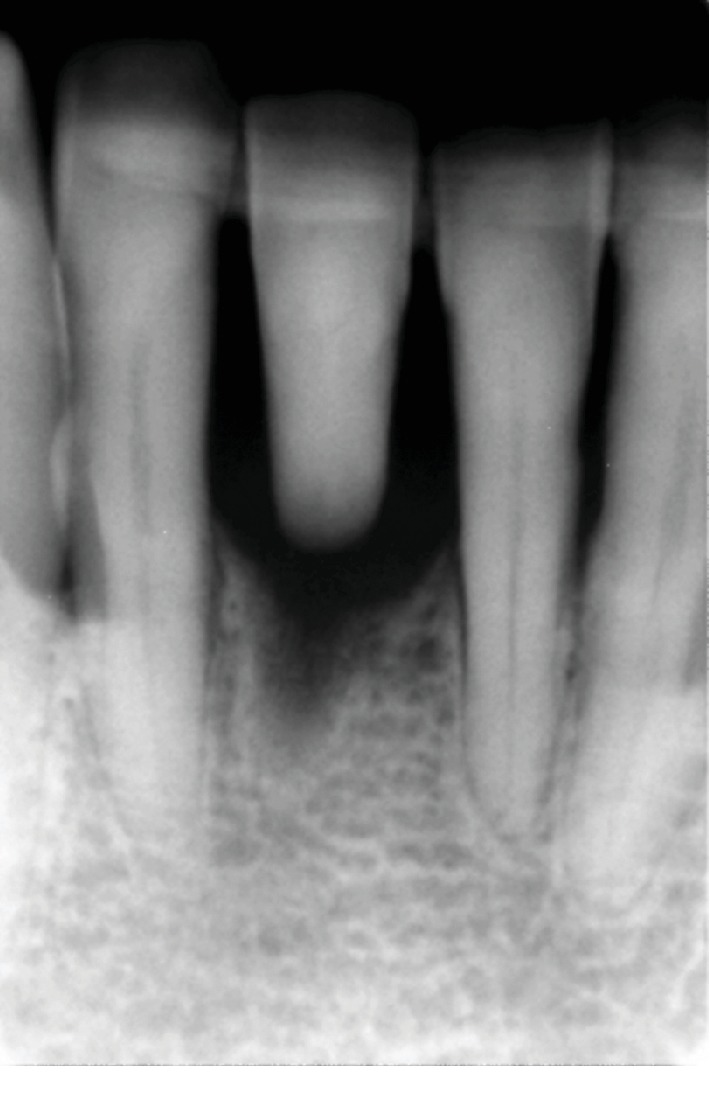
Periapical radiograph showing the natural tooth pontic bonded to adjacent elements; the radiopaque fiber extending to all lower incisors can be appreciated.
